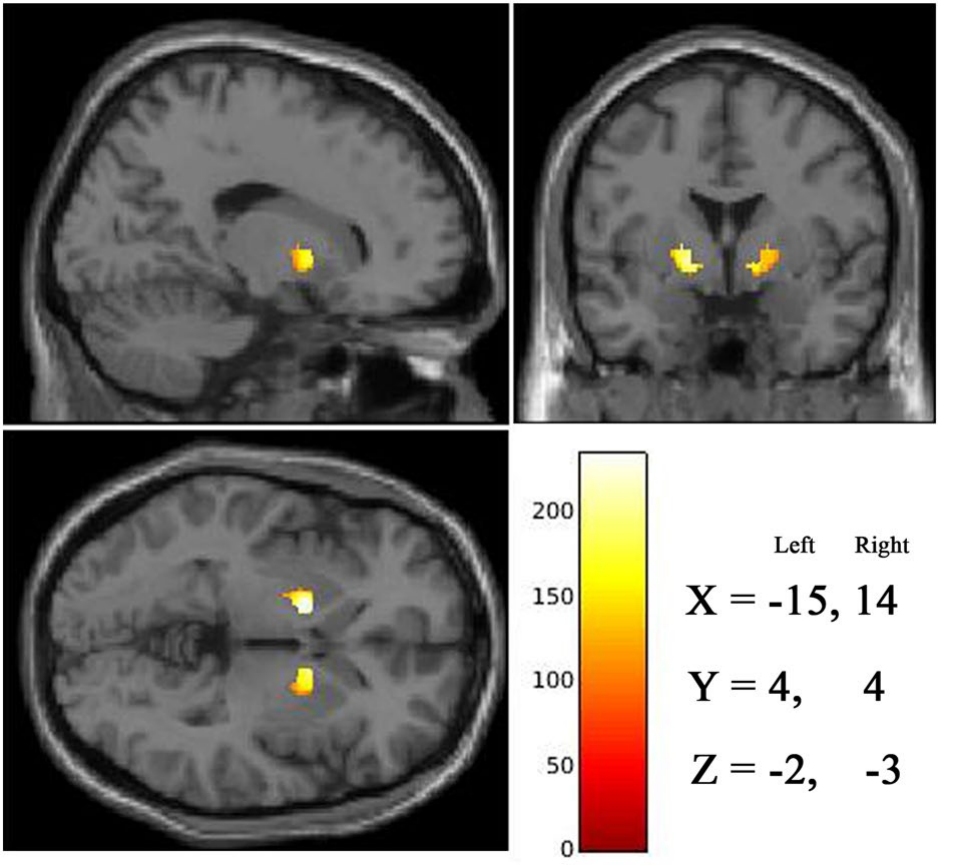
Representative structural MRI image, showing significant (*p* < 0.05 FWE TFCE corrected) positive association of GMV of bilateral ventral pallidum in RM practitioners with hours of meditation practice. Color bar represents TFCE value. These representative structural MRI images were compiled and MNI coordinates were inserted into this compiled image using Adobe Photoshop version CS3 (https://www.adobe.com/in/).
